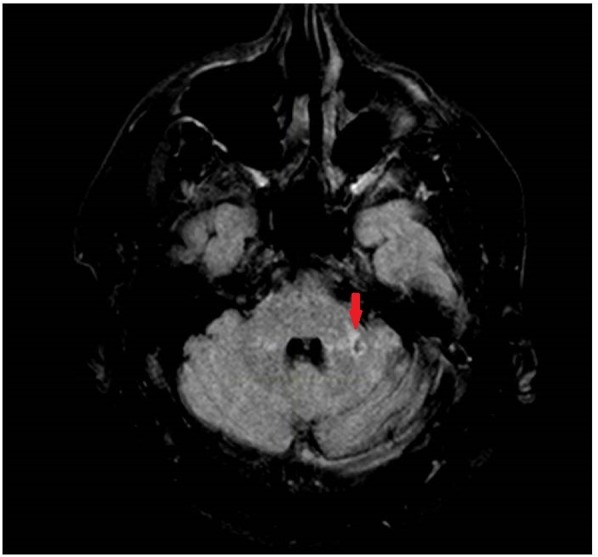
T2 Fluid Attenuated Inversion Recovery (FLAIR) image showing a chronic infarct in the lateral aspect in the left middle cerebellar peduncle.
